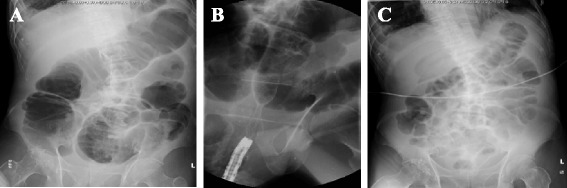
An abdominal x-ray. a Before decompression. b Placement of a metallic stent. c Two days after placement of a metallic stent
